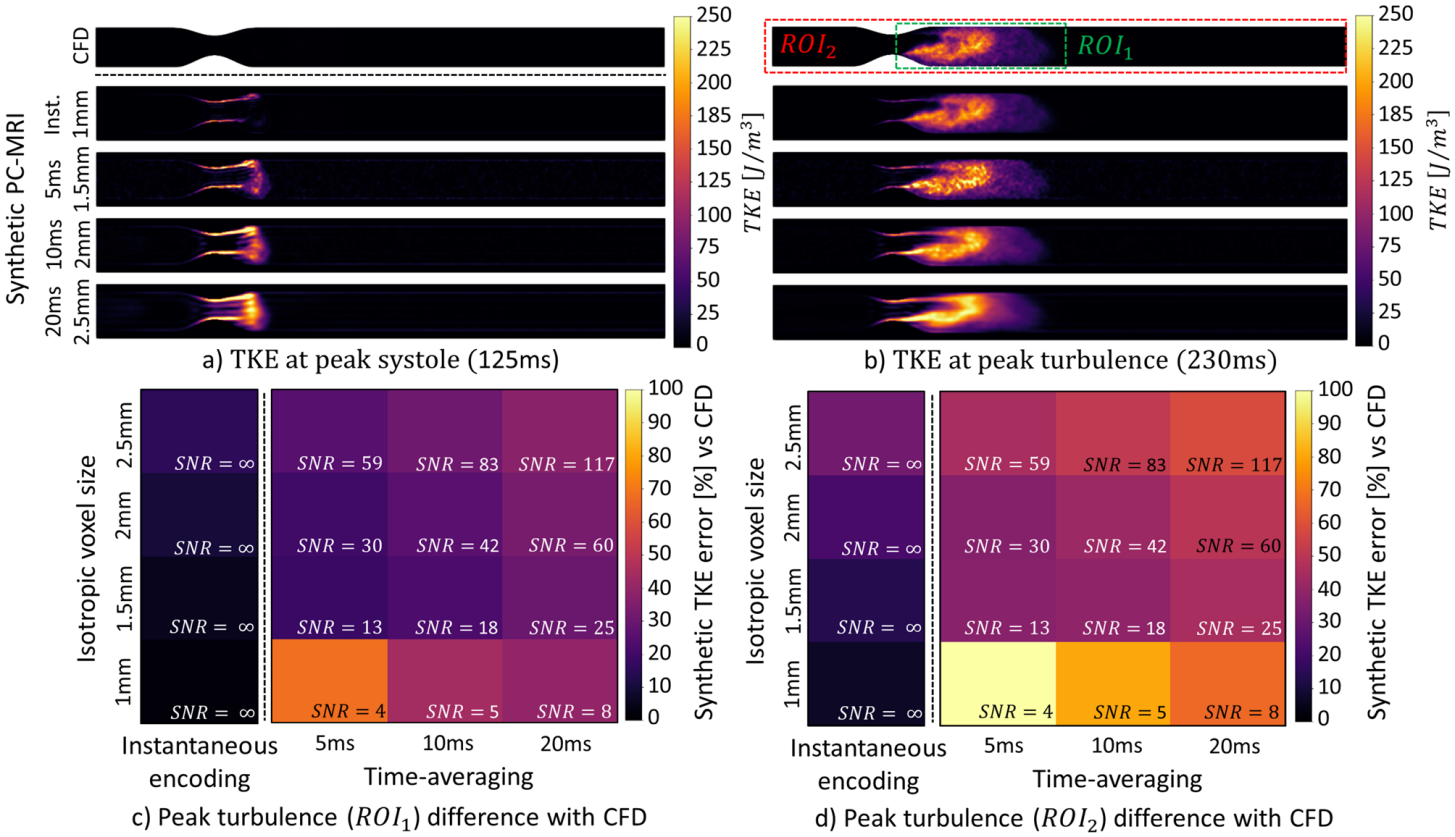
Impact of SNR and spatiotemporal resolution on turbulent kinetic energy for a 75% eccentric stenosis and pulsatile flow. Turbulent kinetic energy at peak systole (a) and at peak total TKE (b) is shown. Percentage errors of peak total TKE are compared in (c) and (d) for $$RO{I}_{1}$$ (envelope of the turbulent region) and $$RO{I}_{2}$$ (whole geometry). Instantaneous (Inst.) encoding refers to a hypothetical noise-free PC-MRI experiment with infinitely high velocity encoding bandwidth.
